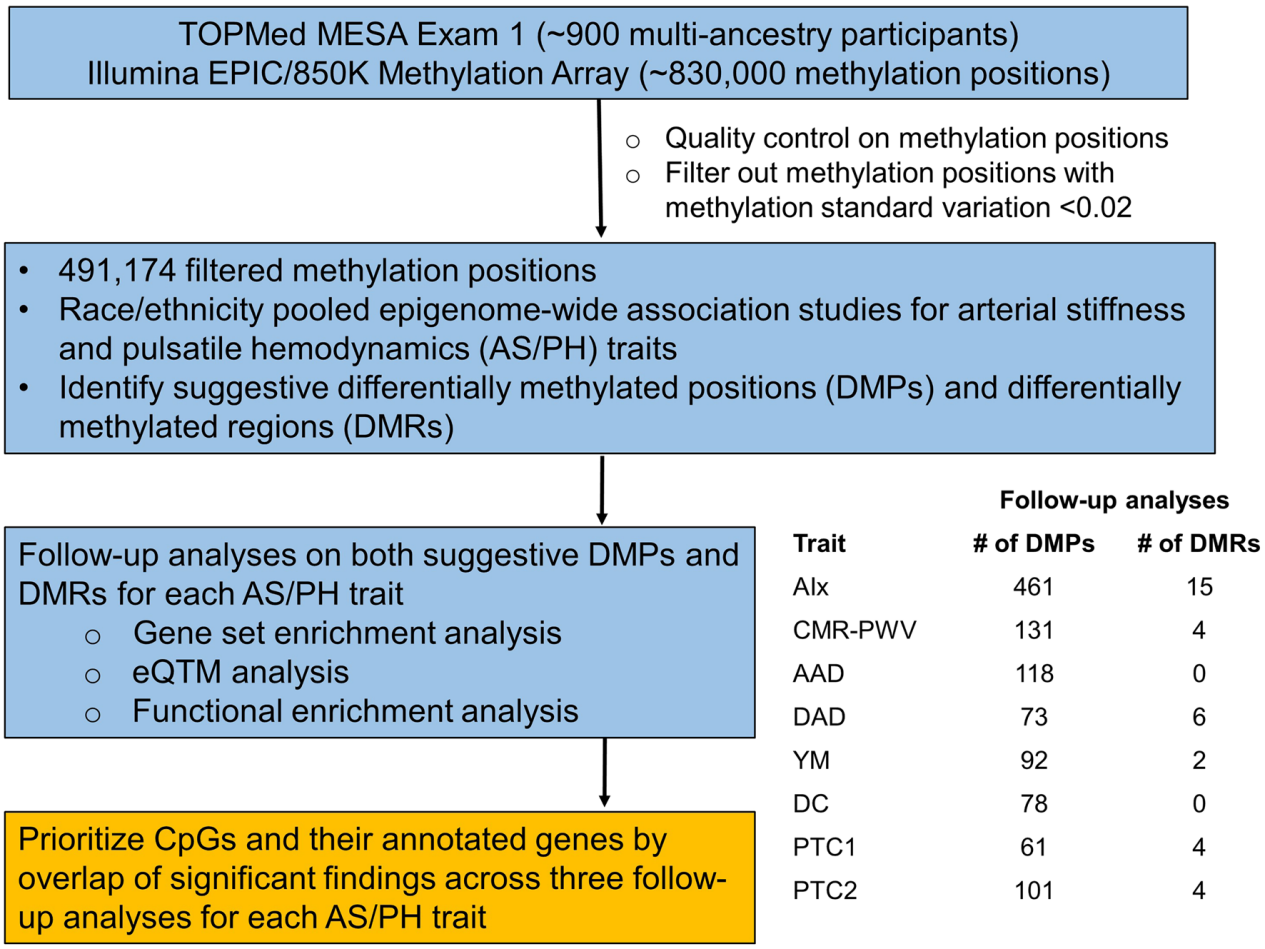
Study design. The suggestive DMP was defined as CpG with association p-value less than 1 × 10^−4^ and 0.05 respectively for epigenome-wide and candidate-gene approaches; the suggestive DMR was defined as co-methylated region with association p-value less than 5 × 10^−4^ and 0.05 respectively for epigenome-wide and candidate-gene approaches; eQTM, expression quantitative trait methylation; AIx, aortic augmentation index; CMR-PWV, aortic arch pulse-wave velocity measured by cardia magnetic resonance imaging; AAD, ascending aortic distensibility; DAD, descending aortic distensibility; YM, Young’s Elastic Modulus; DC, distensibility coefficient; PTC1 and PTC2, radial artery pressure waveform index 1 and 2.
